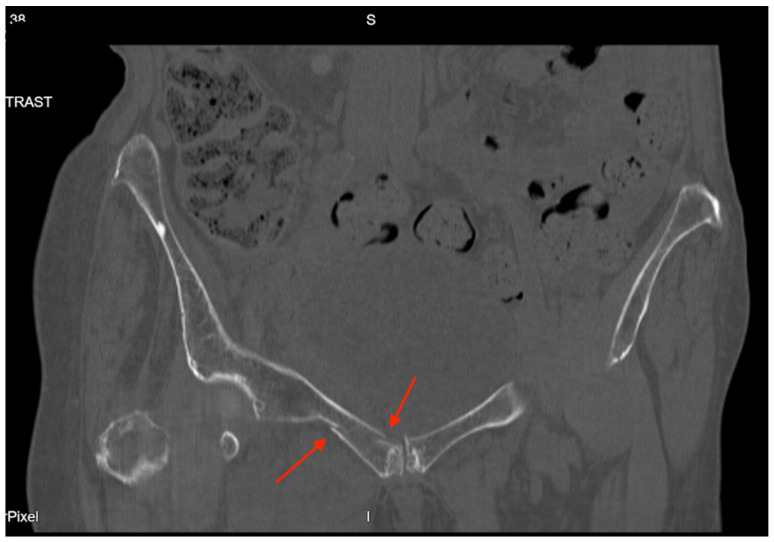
CT abdomen/pelvis images of the described fracture in case 8. Red arrows are used to indicate the location of pelvic fractures for easier identification.
